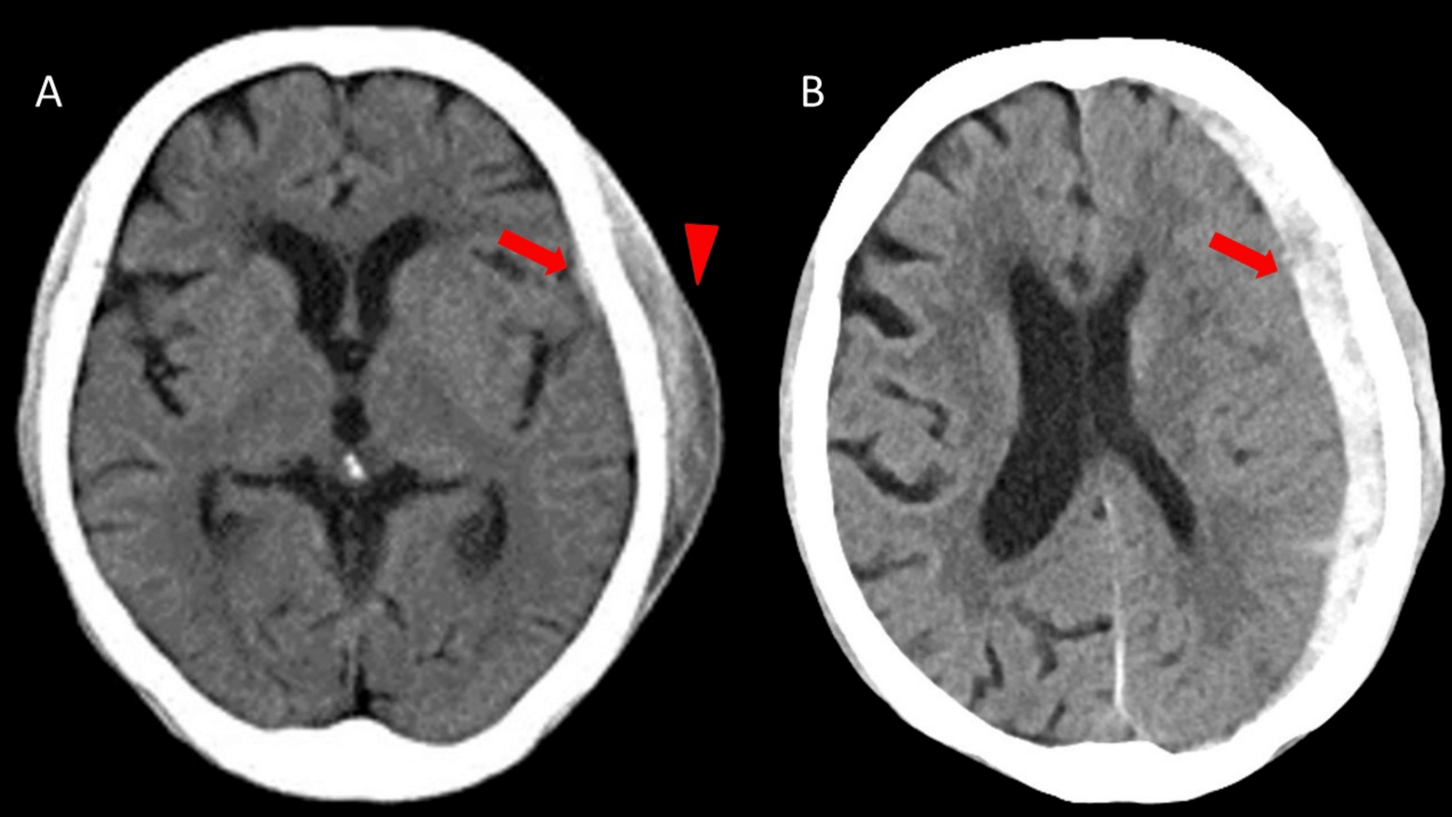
(A) Head CT obtained at another hospital on the day of injury showing a thin acute subdural hematoma (arrow) along the left cerebral convexity and an overlying scalp (subcutaneous) hematoma (arrowhead). (B) Head CT obtained at our hospital seven days after the injury showing enlargement of the acute subdural hematoma (arrow) with increased mass effect on the brain (midline shift).
